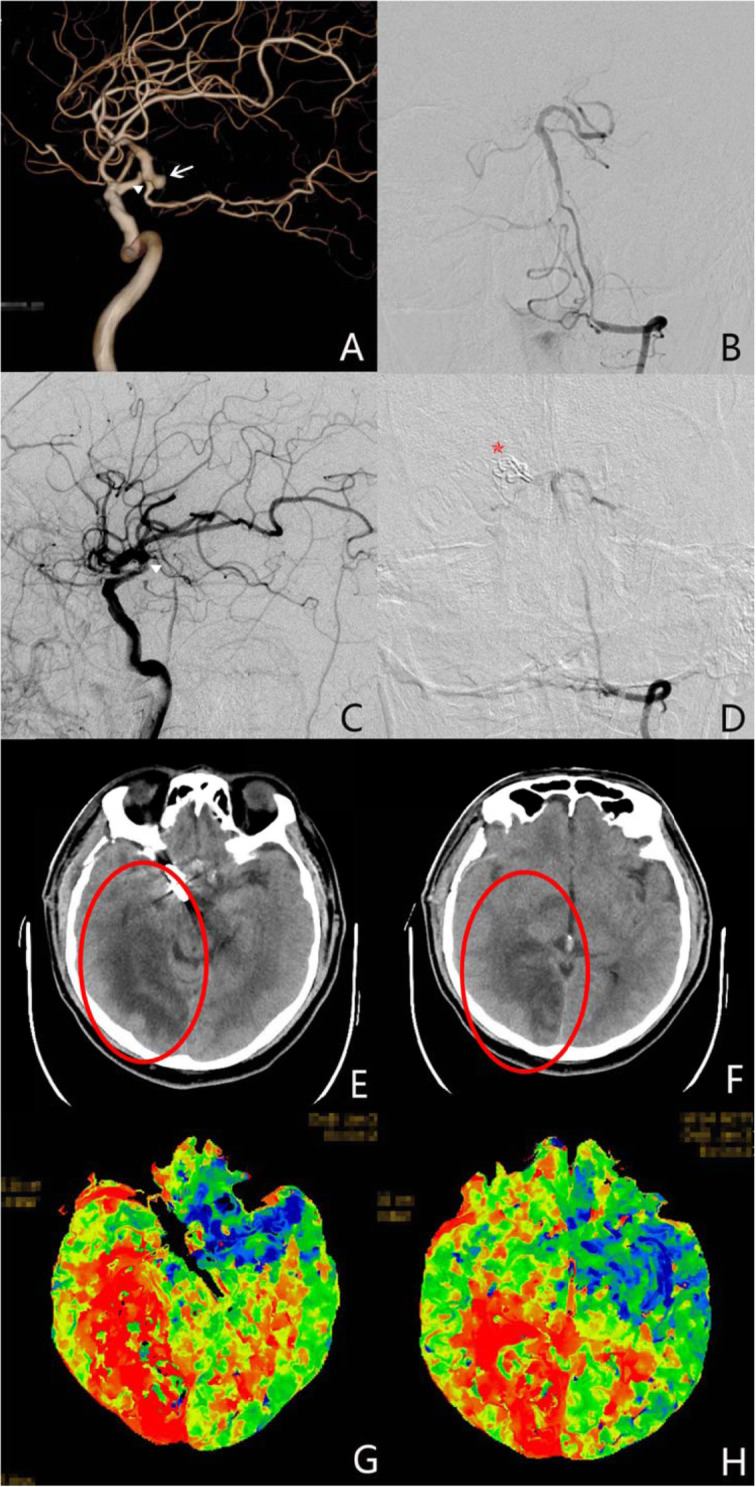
The demonstration of a male, 55-year-old case. a The 3D RCA development of DSA shows the PComA aneurysm originating from the origin of the full-type PCA. White arrowhead shows the aneurysm. The triangle shows the origin of full PCA type from RCA system. b The vertebral development of DSA shows the missing of PCA. c The postoperative RCA development of DSA shows no image of the PCA after surgical clipping and the aneurysm is totally eliminated. The triangle shows the preoperative origin site of full PCA type from RCA system. d The postoperative vertebral development of DSA shows the missing of PCA and totally occlusion of the aneurysm for c. Five-pointed star indicates the titanium clips. e, f The postoperative CT scan show the infarction of right temporal and occipital lobe. The red ellipses demonstrate the ischemic low density. g, h The CT perfusion imaging (axial time to peak) shows the ischemia (red area) of right temporal and occipital lobe
